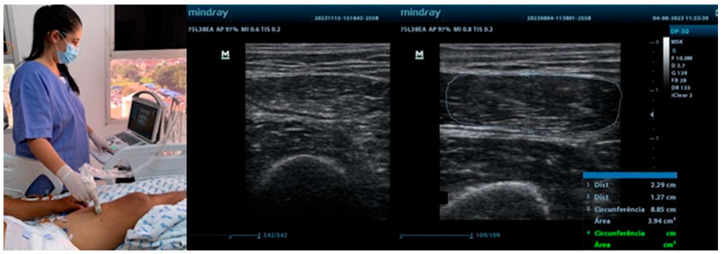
Ultrasound assessment in the ICU.
